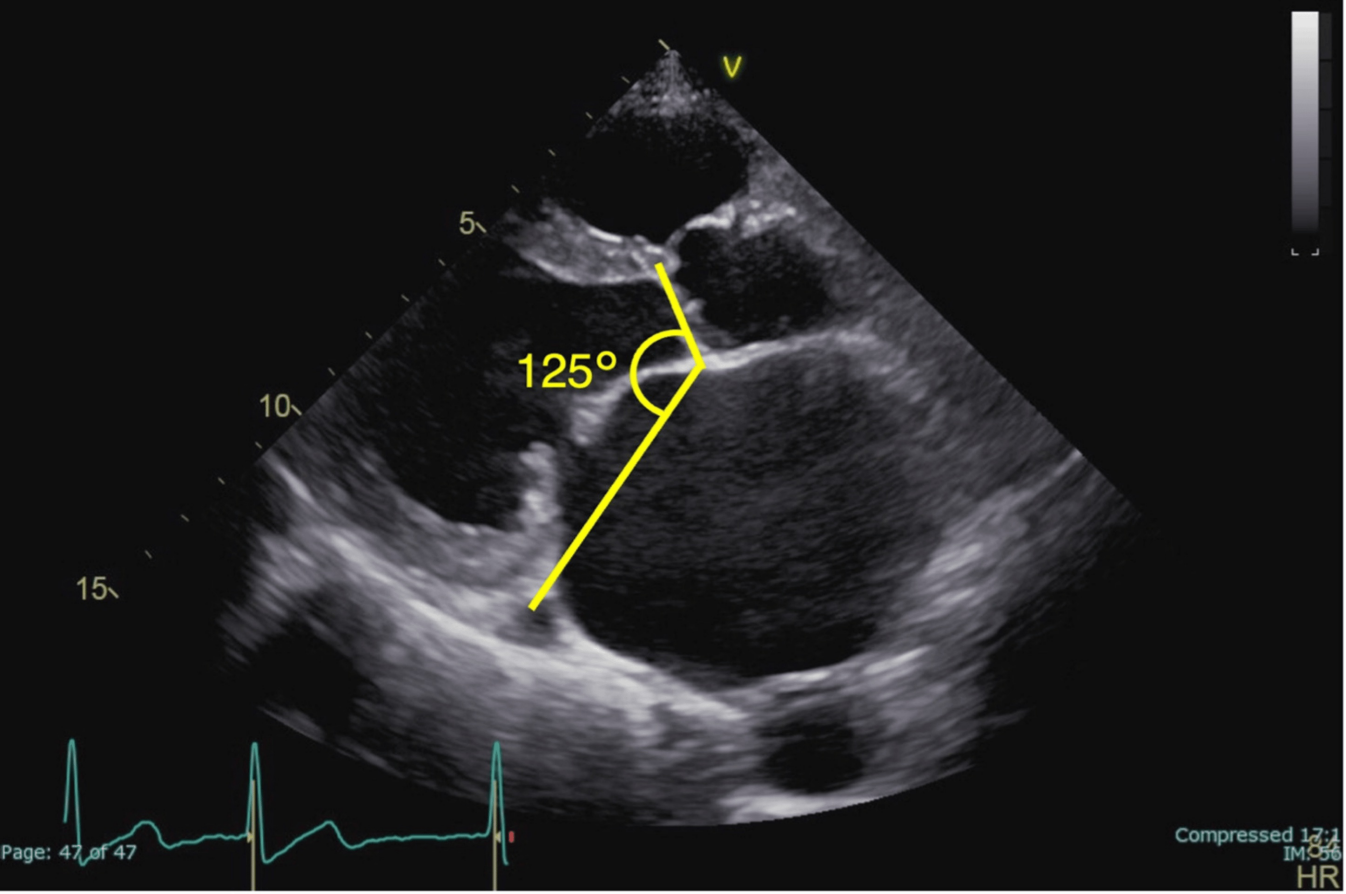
Measurement of the AMA on transthoracic echocardiography AMA: aorto-mitral angle
